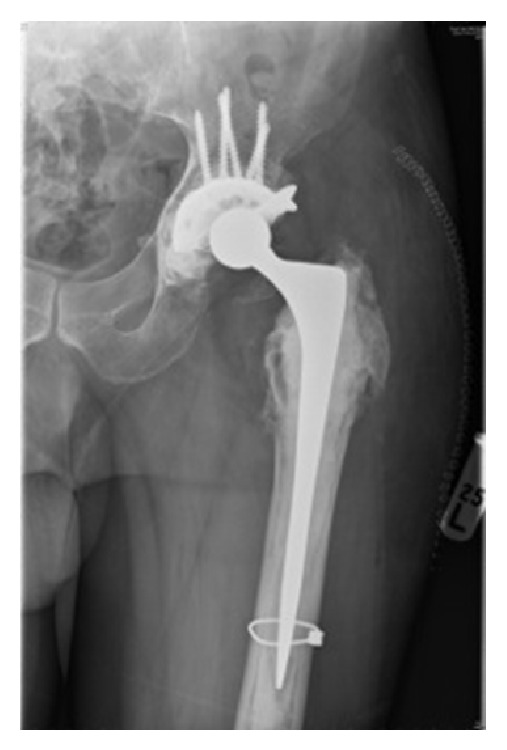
Postoperative radiograph of cemented CPT size 4 extended offset stem.
